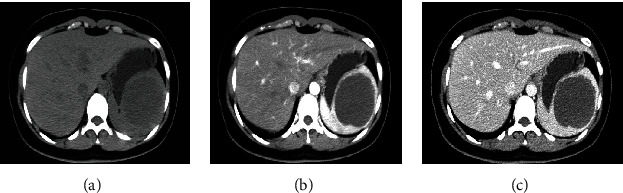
Abdominal CT-enhanced images: (a) shows plain scan; (b) shows arterial phase; and (c) shows venous phase. The upper part of the spleen is about 7-8 cm with slightly low-density shadow.
